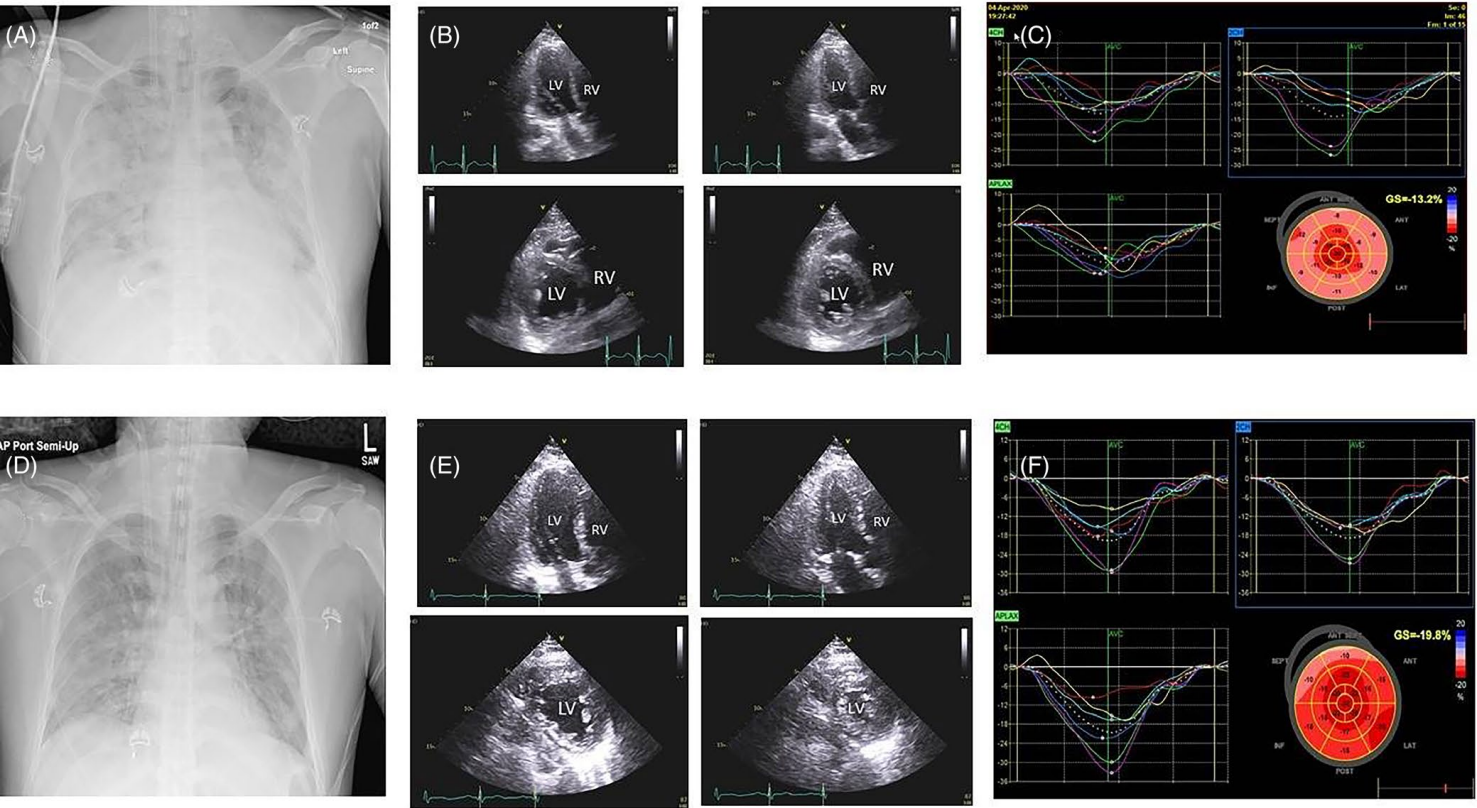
Panel A. Baseline chest X‐ray AP view showing diffuse lung parenchymal opacities consistent with acute respiratory distress syndrome. Panel B. A baseline 2D transthoracic echocardiographic (TTE) apical 3‐chamber view in end‐diastole (upper left image), end‐systole (upper right image), and parasternal short‐axis view at midventricular level in end‐diastole (lower left image) and end‐systole (lower right image). Increased left ventricular (LV) end‐systolic cavity size from basal to mid segments is shown (Video S1). Panel C. 3‐, 2‐, and 4‐chamber strain maps and bull's eye plot showing regional and global averaged LV longitudinal peak systolic strain of ‐ 13.2% with an apical sparing pattern. Panel D. Follow‐up chest X‐ray AP view showing marked improvement in ARDS. E. Follow‐up 2D TTE shows an apical 3‐chamber view in end‐diastole (upper left image), end‐systole (upper right image), and parasternal short‐axis view at midventricular level in end‐diastole (lower left image) and end‐systole (lower right image). The change of LV chamber size over the cardiac cycle suggests normalization of wall motion and LV ejection fraction (Video S2). Panel F. Follow‐up regional strain maps and global averaged LV longitudinal peak systolic strain of −19.8% with near‐normal strain pattern
